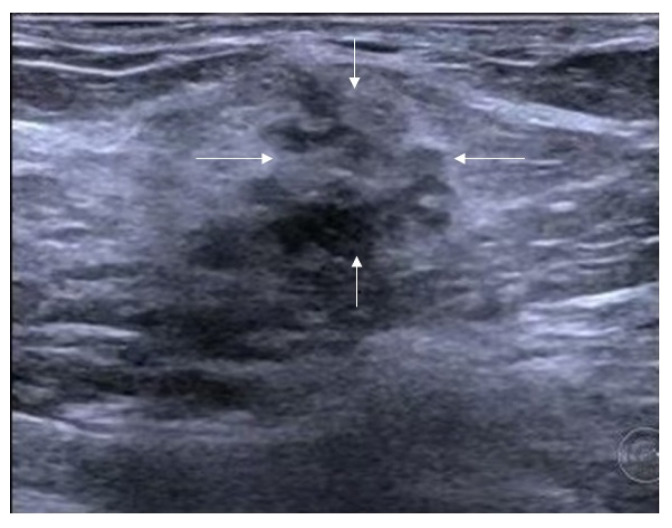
Ultrasound features of non-mass breast lesions of Type III. A case of DCIS. Ultrasound shows focal contraction/distortion of breast tissue at localized area within the breast (indicated by the white arrows).
